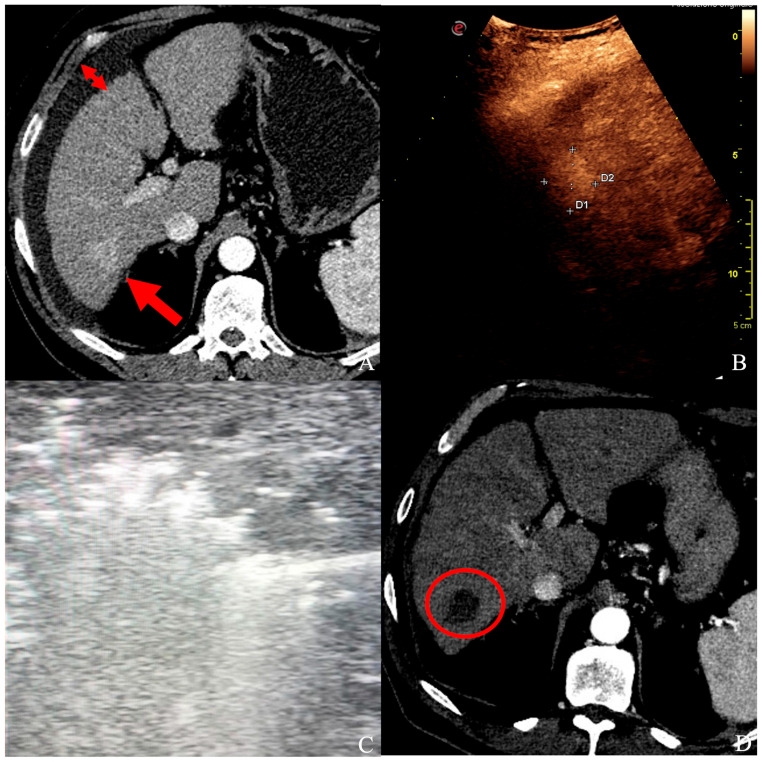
(A) A patient with ascites (short arrows) and a 25 × 20 mm HCC in segment VI (long arrow), confirmed by contrast-enhanced ultrasound (B), and unsuitable for ultrasound-guided percutaneous ablation. (C) Under laparoscopy, HCC was ablated with a single probe deployment, and the whole nodule turned hyperechoic. (D) At follow-up CT control, a well-defined necrotic volume with no signs of HCC persistence in segment VI (red circle).
